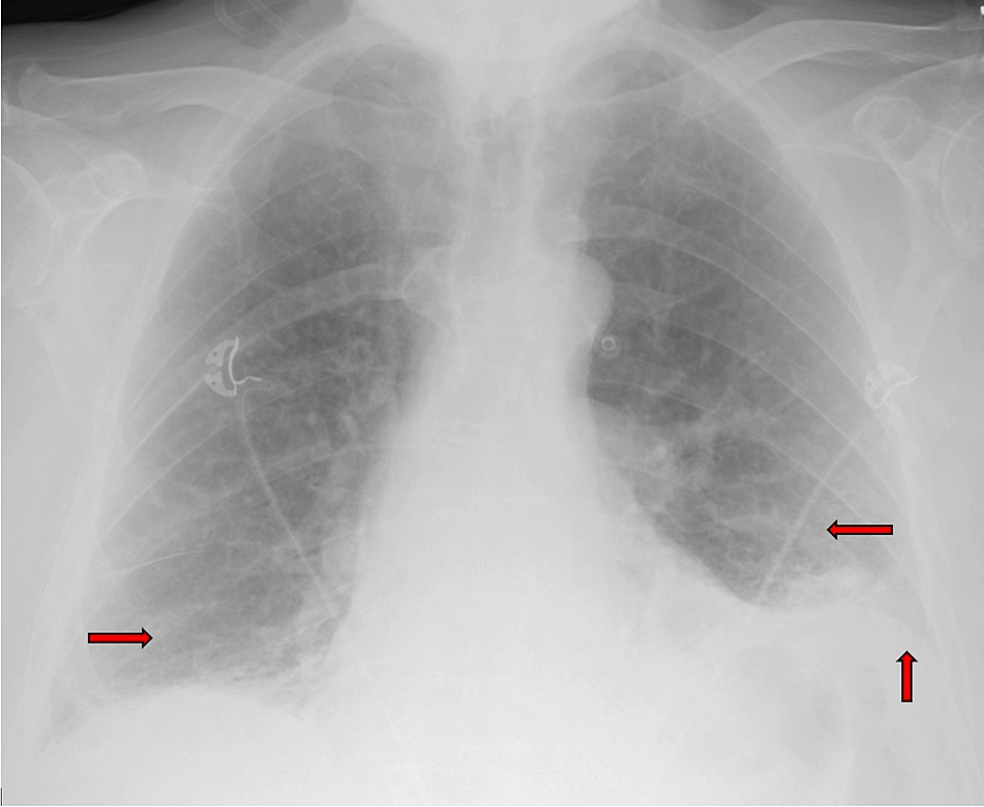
A portable chest X-ray revealed bibasilar infiltrates (horizontal red arrows) and left trace pleural effusion (vertical red arrow).
